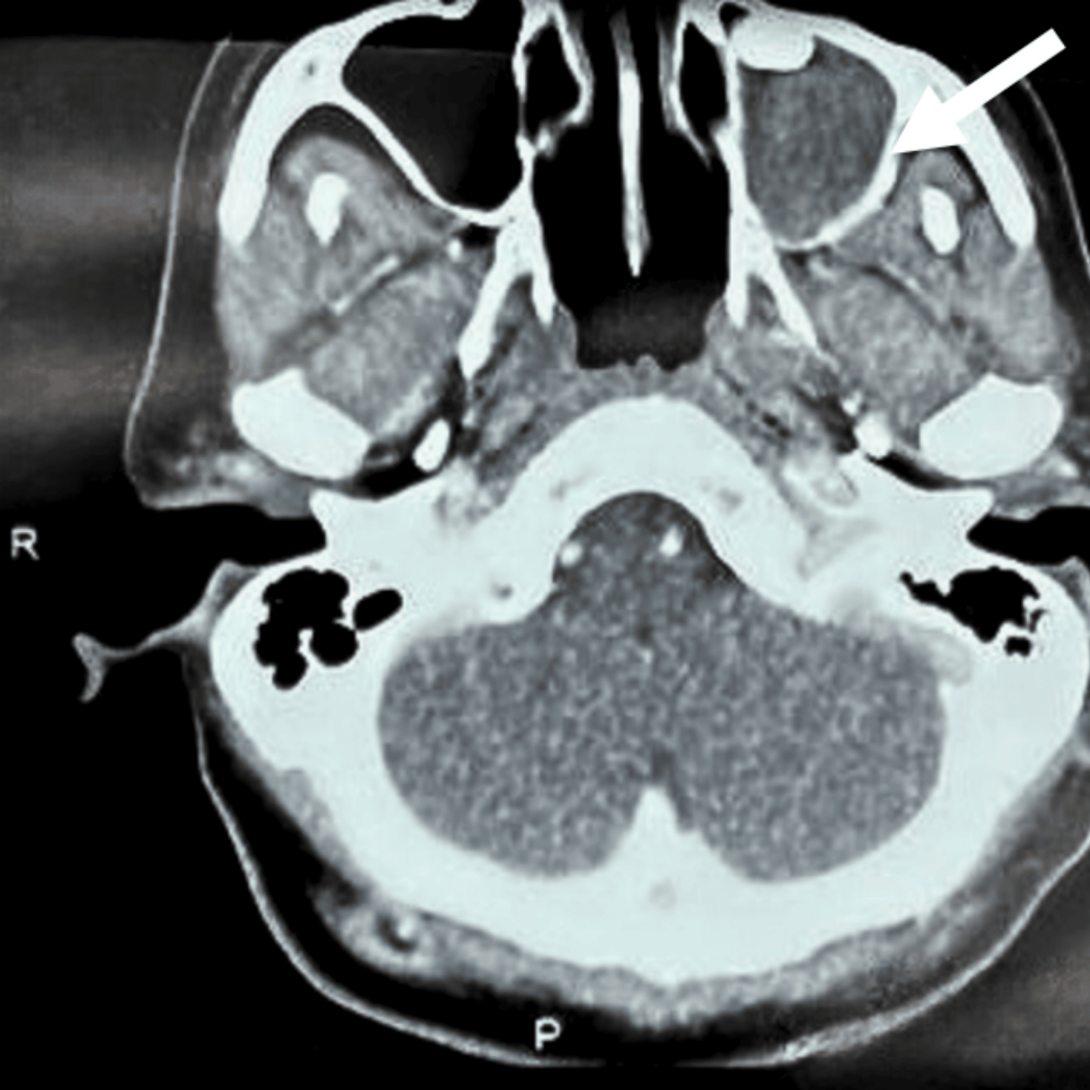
The CT scan (axial view) shows cortical defects over the lateral (white arrow) and inferior aspects of the left maxillary sinus.
